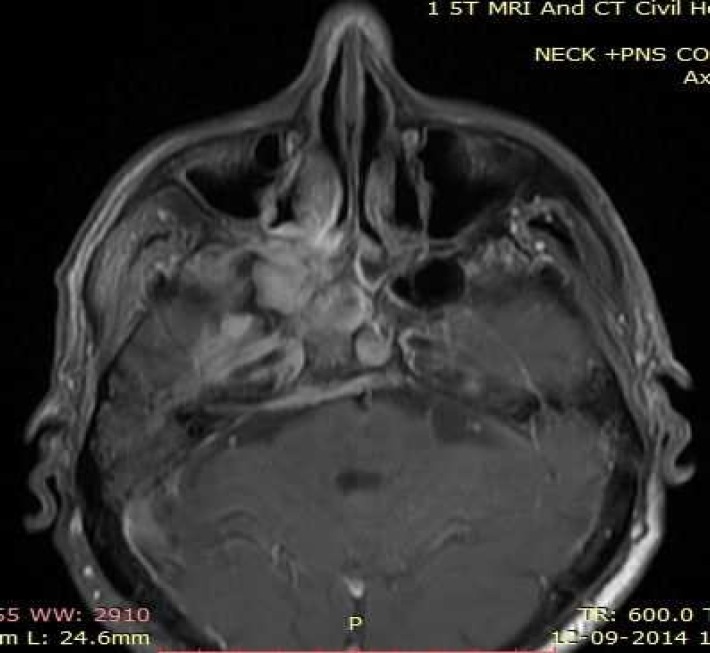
A gadolinium enhanced magnetic resonance imaging of the head and neck region revealed primary nasopharyngeal mass lesion of size 49 × 62 × 47 mm
